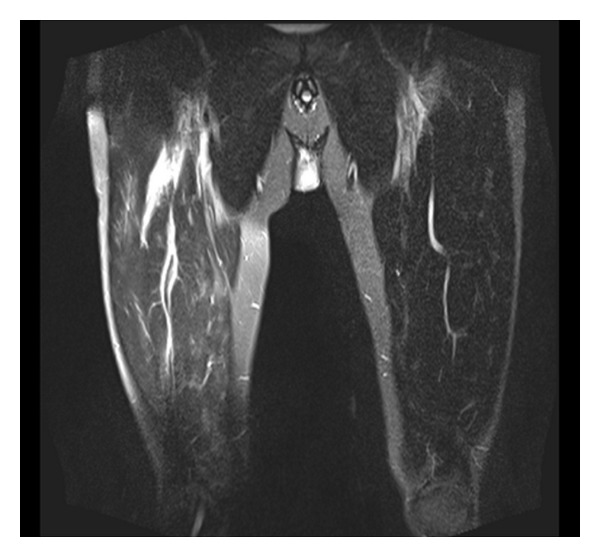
Coronal STIR MRI image showing high signal change in the right thigh associated with intramuscular oedema.
